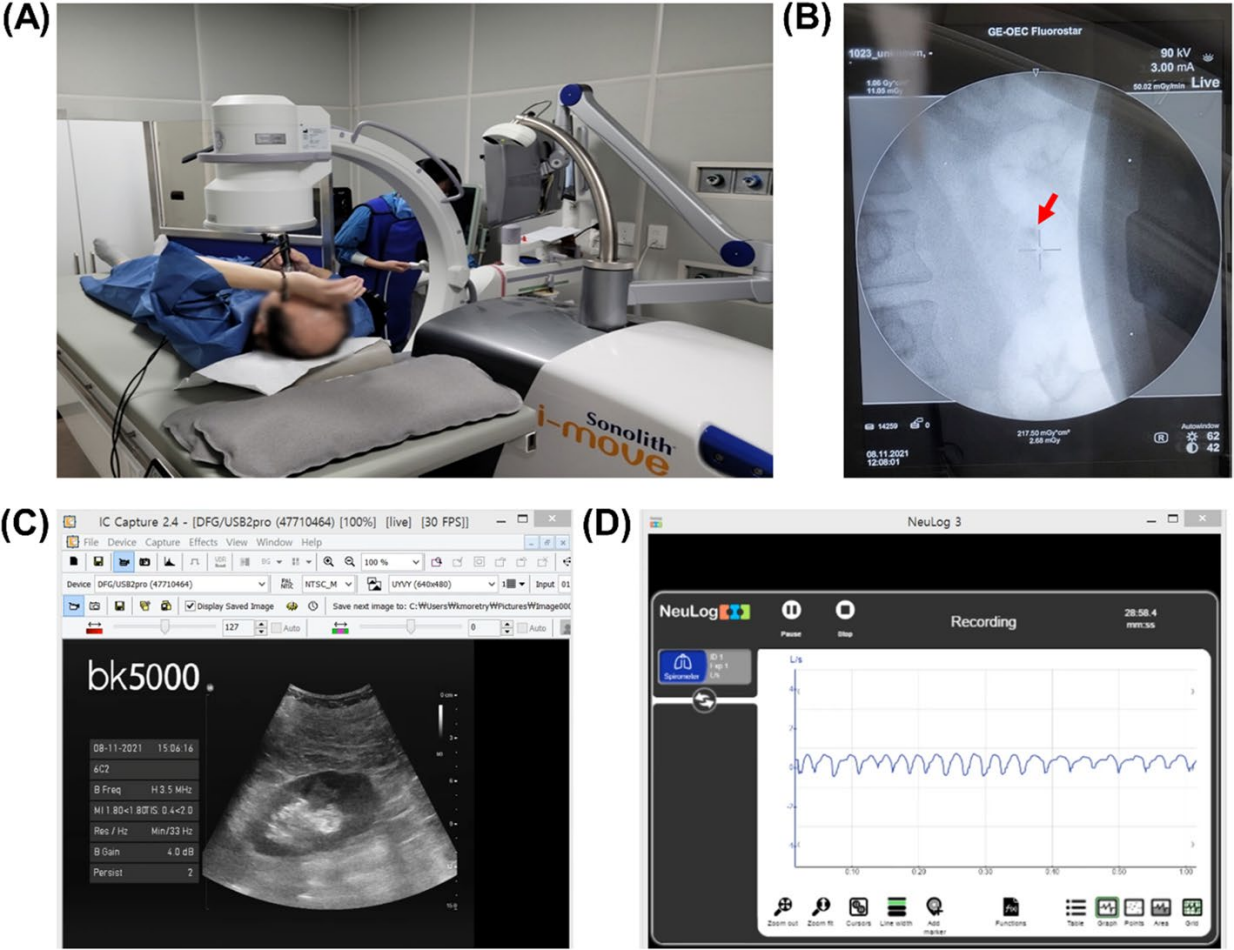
Evaluation of respiration. a, b The position of the bed was set to fit the shockwave using X-rays, the operator explained to the patient the reason for measuring respiration during lithotripsy, then lithotripsy began. c, d Patients’ respiration curves of inhalation and exhalation were recorded, and the locations of the stones in the kidney were predicted using ultrasound to recognize a specific breath point and generate high-accuracy shockwave targeting
